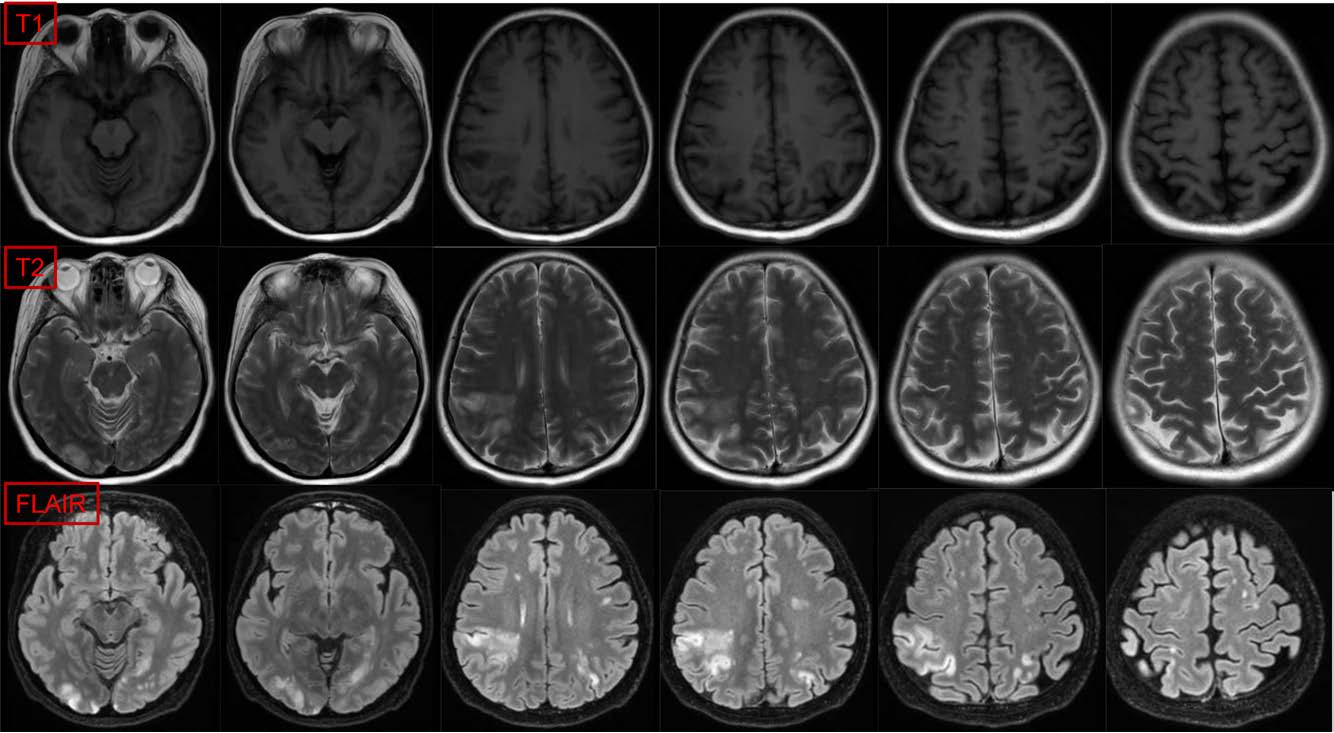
Brain MRI revealed multiple acute cerebral infarctions in bilateral cerebral and cerebellar hemispheres, especially in the right parietal lobe (T1, T2, FLAIR). MRI = magnetic resonance imaging.
